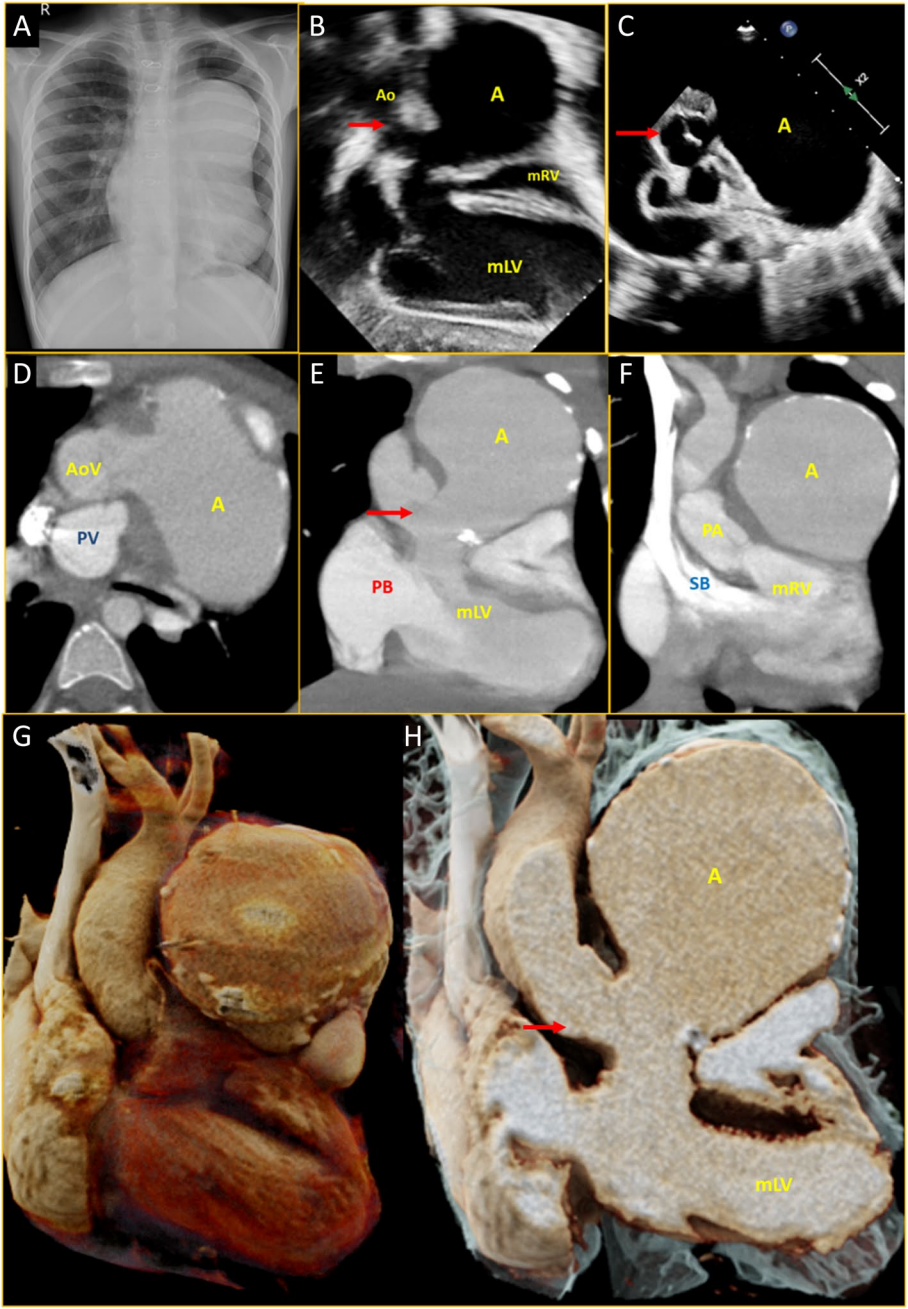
A Three-year post-operative chest X-ray showing huge para-cardiac mass. B Transthoracic echocardiogram subxiphoid view showing right sided morphological left ventricle connected to aorta with huge aneurysm just beneath the valve (red arrow). C Basal short axis view showing aortic valve with huge aneurysm arising from LVOT just below the aortic valve (red arrow). D Cardiac CT axial section showing aorta is anterior to pulmonary artery and aneurysm is sub-valvular. E Cardiac CT in coronal section showing right atrium with pulmonary baffle communicating with right sided morphological left ventricle, in turn connected to aorta with huge sub-valvular (aortic valve: red arrow) LVOT tunnel aneurysm. F Coronal section showing systemic baffle connected to left sided morphological right ventricle in turn connected to pulmonary artery. Note that: before Senning operation, left atrium was connected to left sided right ventricle, confirming ventricular inversion. G, H Volume rendered technique showing the spatial relationship of the aneurysm. A: aneurysm, Ao: aorta, AoV: aortic valve, CT: computed tomography, LVOT: left ventricular outflow tract, mLV: morphological left ventricular, mRV: morphological right ventricular, PB: pulmonary baffle, PV: pulmonary valve, SB: systemic baffle
